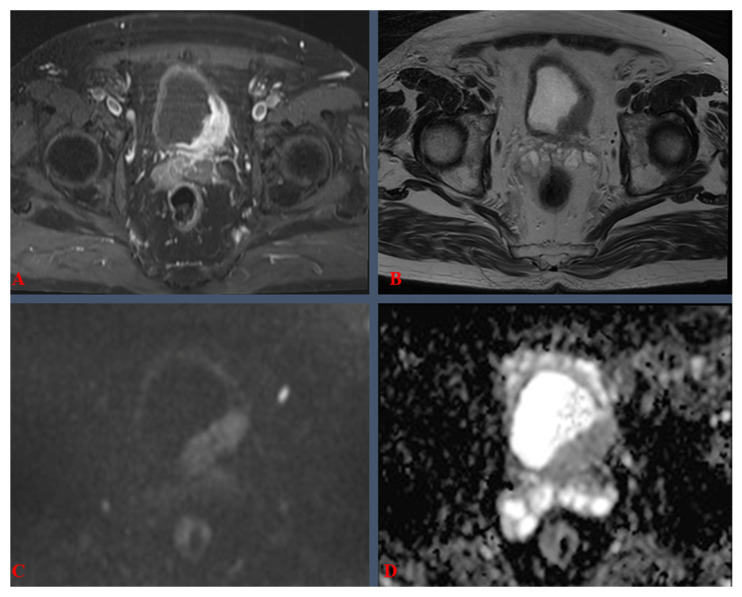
Multiparametric magnetic resonance imaging of the pelvis, at initial presentation of a bladder tumor case, scored as VI-RADS 4, on a 1.5-Tesla machine: (A) post-contrast fat-saturated axial T1-weighted sequence; (B) axial T2-weighted sequence; (C) diffusion-weighted imaging (DWI), axial sequence; (D) apparent diffusion coefficient (ADC) axial map. The mean ADC value for this tumor was 0.85 × 10^−3^ mm^2^/s.
